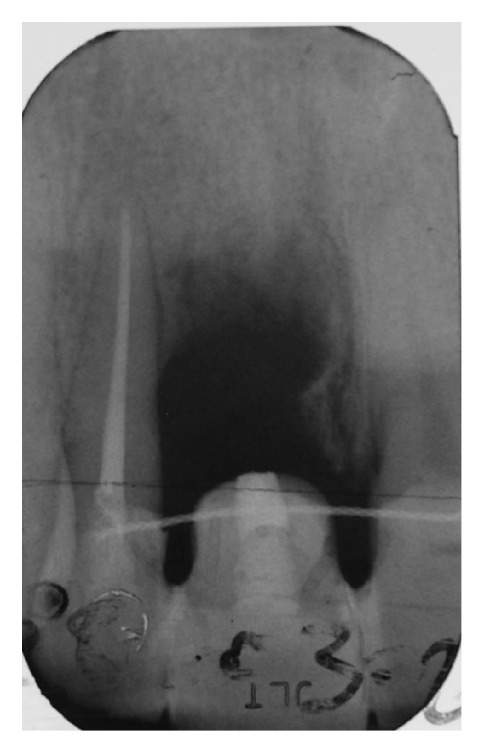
The radiograph taken before implant insertion shows a complete loss of the buccal and palatal bone.
